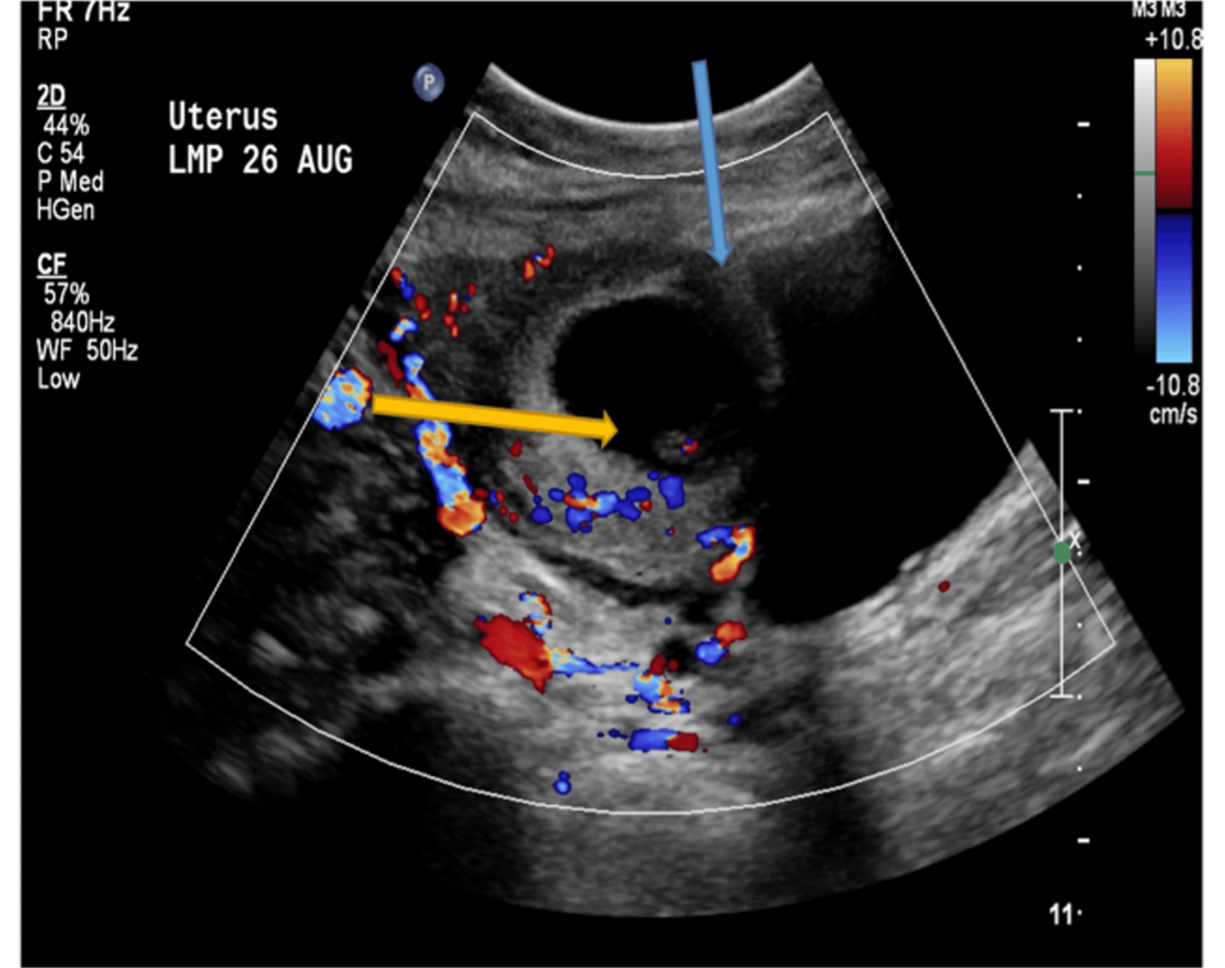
Axial transabdominal ultrasound demonstrating the characteristics of cesarean scar ectopic pregnancy Thin myometrium between gestational sac and urinary bladder (the blue arrow). The gestational sac contains a fetal pole with a positive heart color (the yellow arrow).
